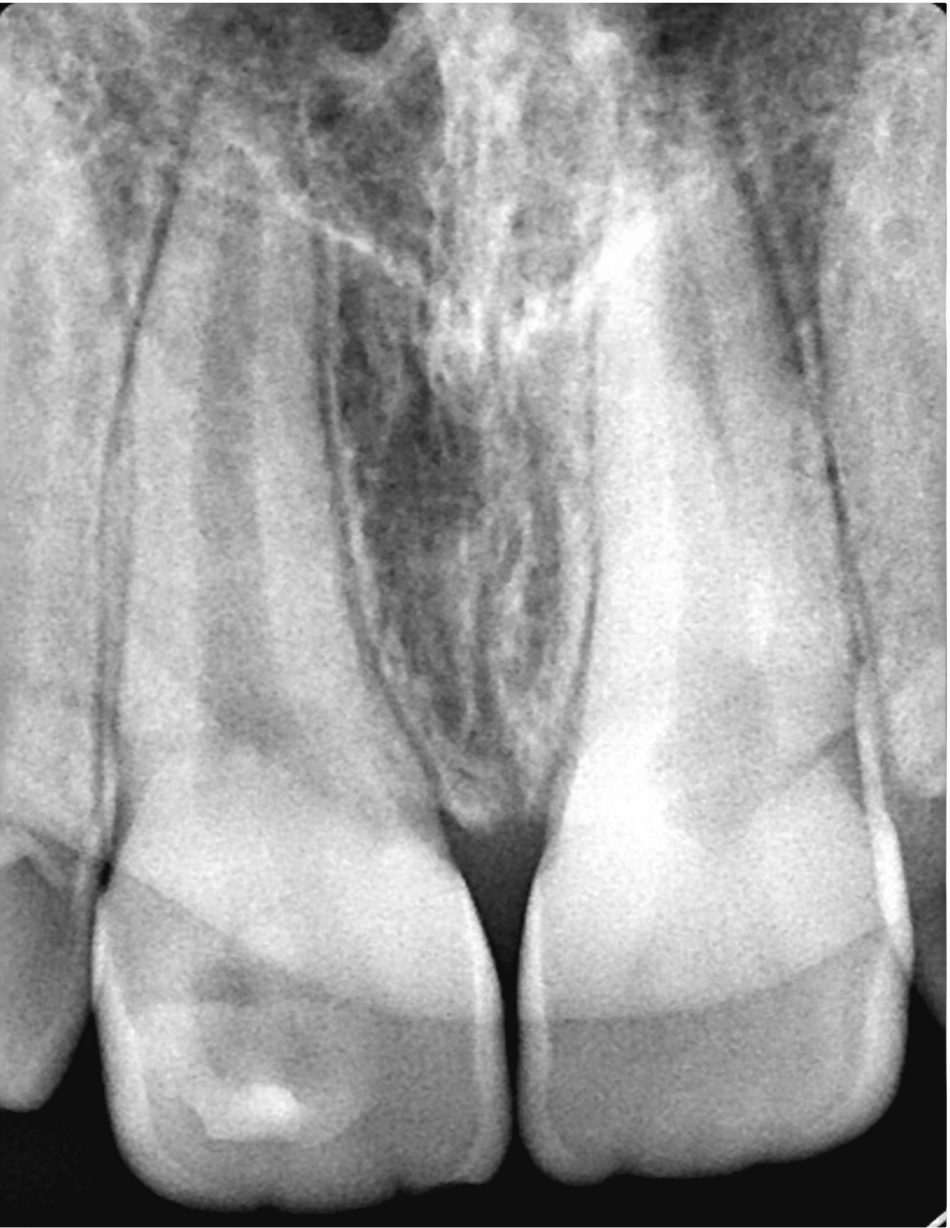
Periapical radiograph showing the closed apex of #11
